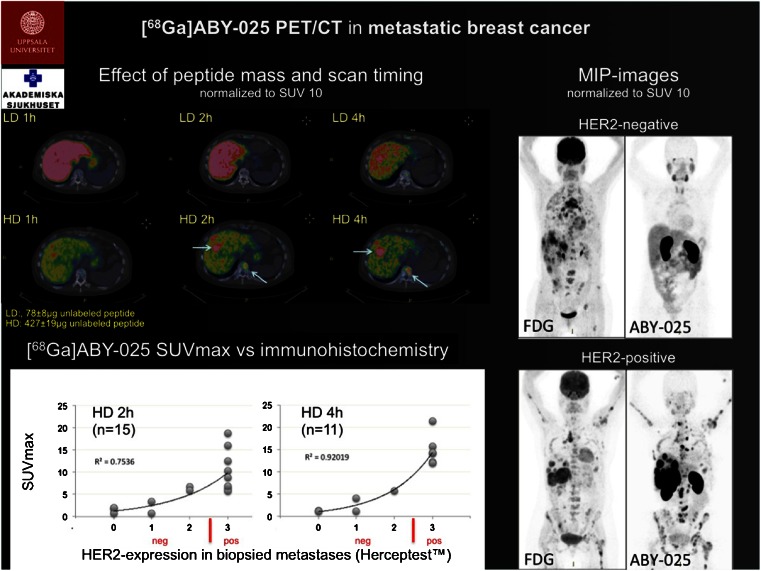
^68^Ga-ABY025 PET/CT imaging in metastatic breast cancer [43]
